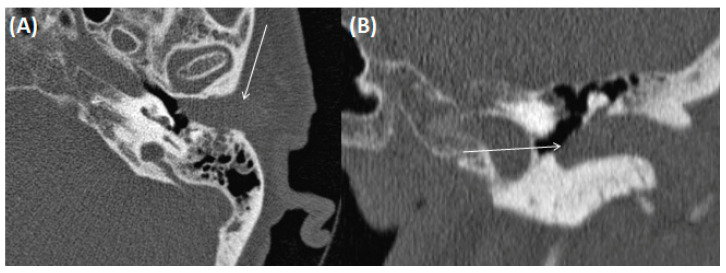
CT of a 73-year-old female patient in axial (A); and coronal orientation (B). Due to complete soft tissue obstruction of the external auditory canal, otitis externa maligna was assumed. However, after biopsy a squamous cell carcinoma was confirmed.
